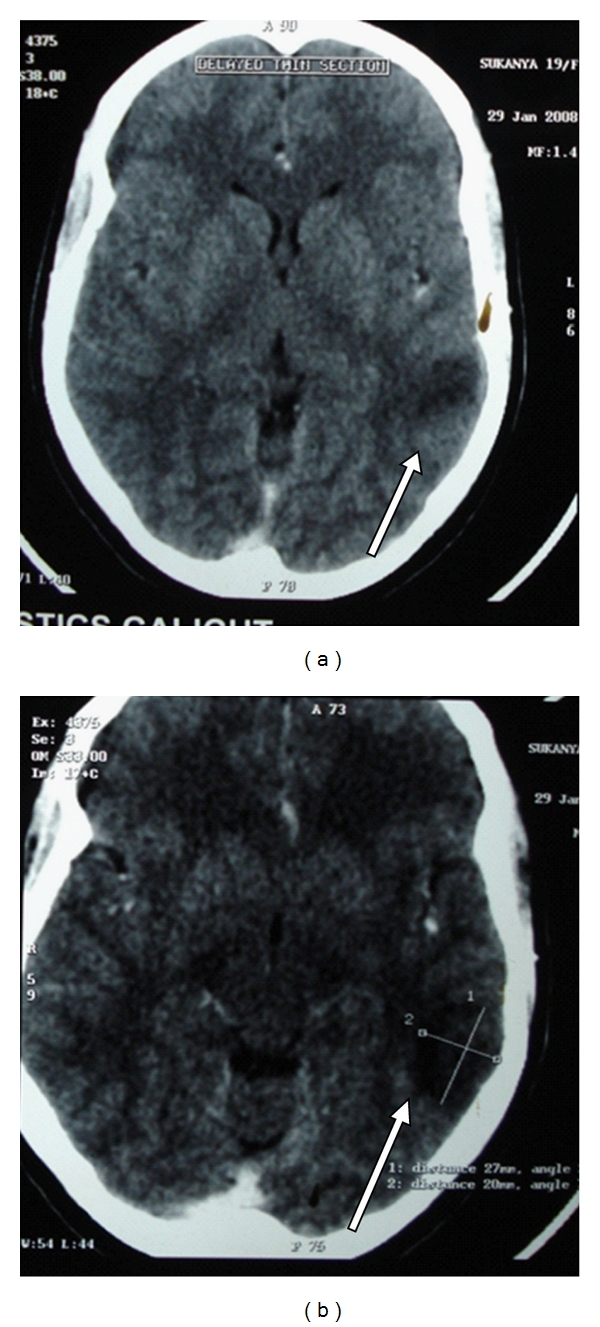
Plain CT scan showing hypodense lesion mistaken for glioma (actually venous infarct).
